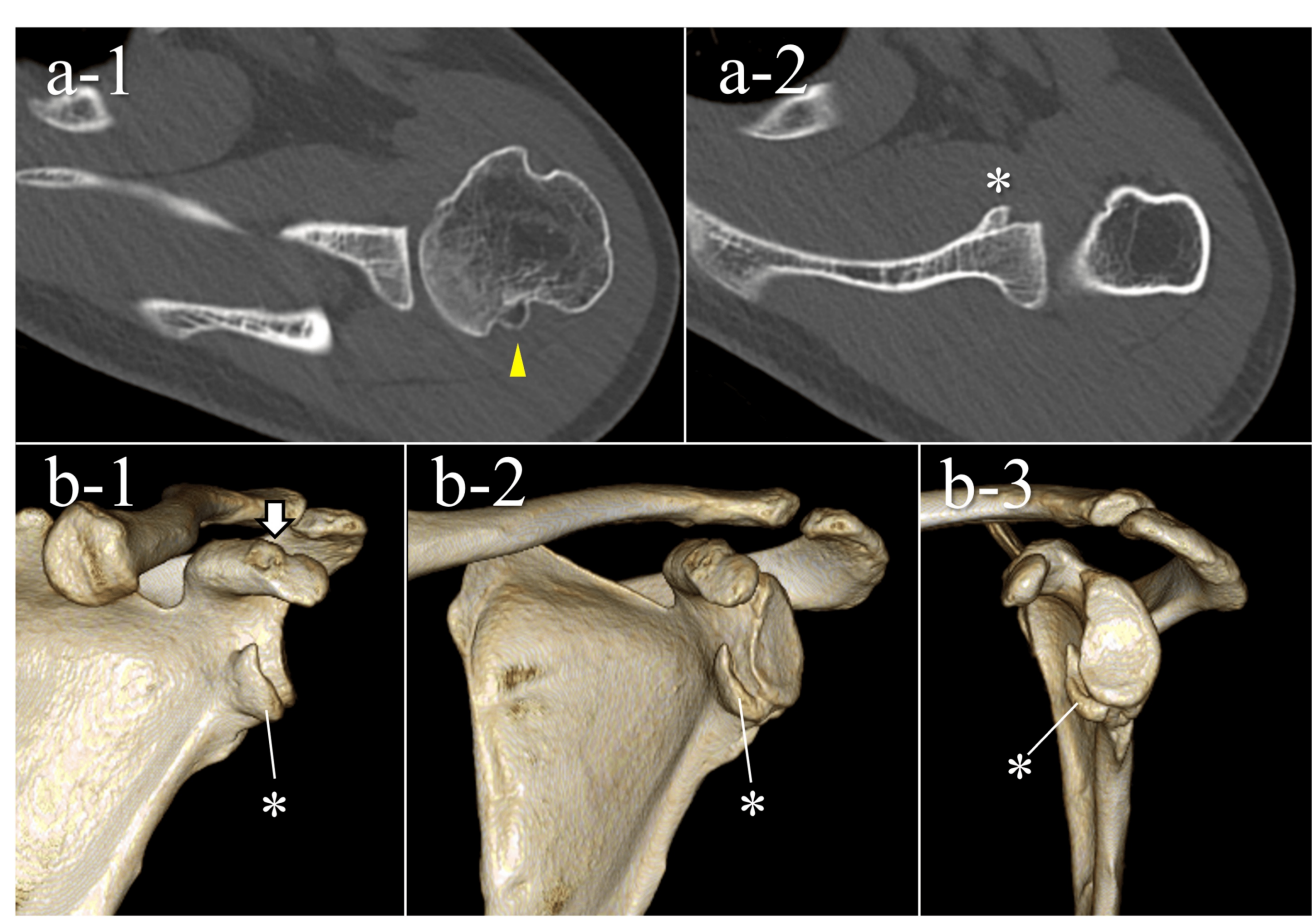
CT findings at one year after injury Axial CT images show bony union of the separated fragment within the Hill-Sachs lesion (a-1, arrowhead) and healing of the bony Bankart lesion deep inside the glenoid (a-2, asterisk). Three-dimensional CT reconstructions demonstrate bony union of the coracoid process (b-1, arrow) and the bony Bankart lesion (b-1,2,3, asterisk). CT, computed tomography
